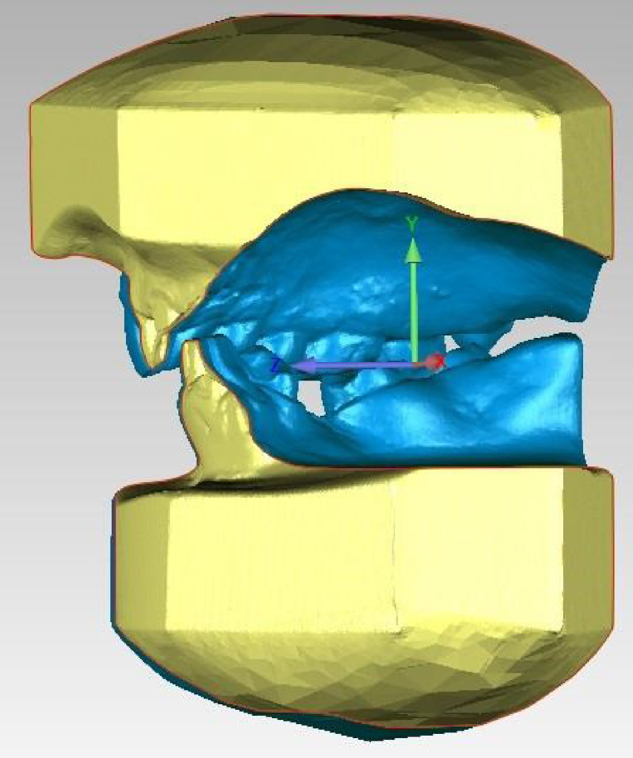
Measurement of Overjet (Plane Z) and Overbite (Plane Y) on digital model.-Amount of overlapping of anteroinferior teeth by the anterosuperior teeth (overbite): measured from the amount of vertical overlap of the incisal border of the central upper incisor to the incisal border of the more extruded lower central incisor in millimeters, vertically,^31^ represented by plane Y (Fig. 7);-The angulation of the lower incisors was assessed in the lateral cephalometric radiographs taken before and after treatment, which in the case of this study, were analyzed through Tweed's IMPA angle,^32^, ^33^ consisting of the mandibular plane, traced using as reference a plane tangential to the lower border of the mandible and the long axis of the lower incisor.
